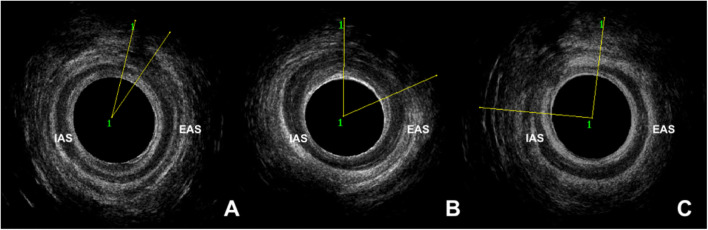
Endoanal ultrasonography findings of three different women 3 months following primary repair OASI. a Endoanal ultrasonography showing a scar within the external anal sphincter measuring 20 degrees, equivalent to a defect ≤1 hour (h). b Endoanal ultrasonography showing an external anal sphincter defect measuring 63 degrees, equivalent to a defect of approximately 2 h in size. c Endoanal ultrasonography showing an internal and external anal sphincter measuring 96 degrees, equivalent to a defect of approximately 3 h in size
